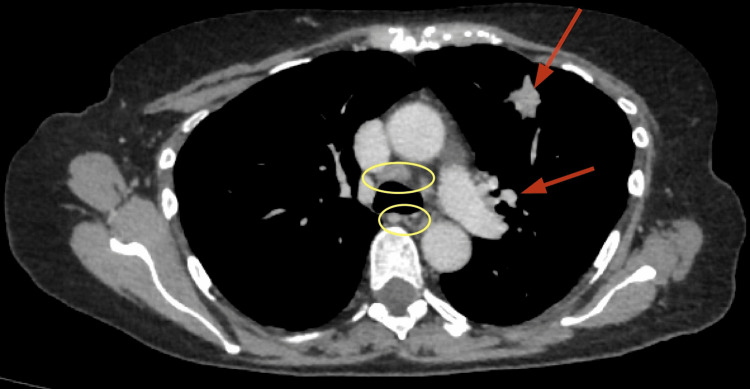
Contrast-enhanced CT chest showing multifocal consolidation and mediastinal lymphadenopathy on initial presentation Axial contrast-enhanced CT of the chest demonstrating multifocal pulmonary consolidation (red arrows) and enlarged mediastinal lymph nodes (yellow circles) on the mediastinal window. These findings were consistent with an inflammatory or infiltrative process, prompting exclusion of infection and further haematological workup.
